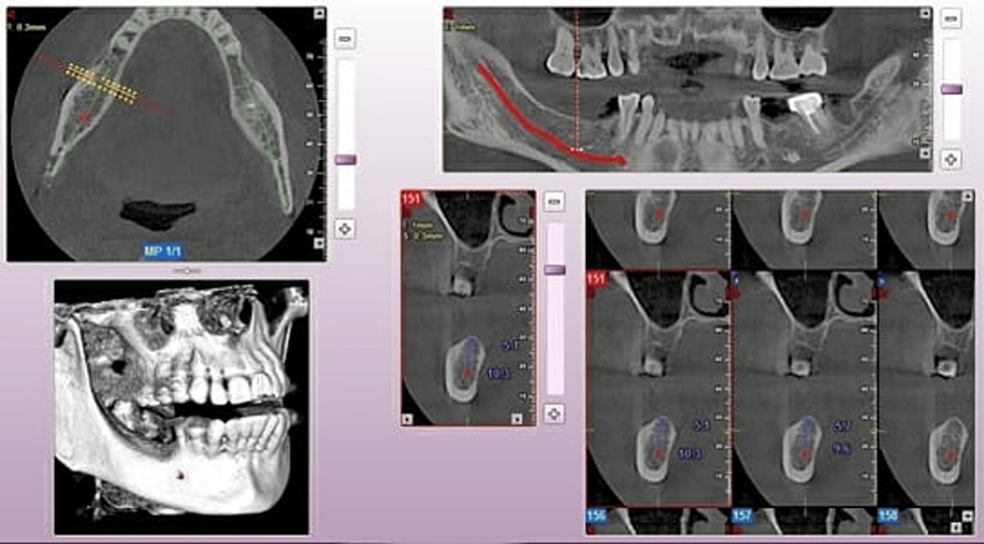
Pre-operative computer tomography (CT) image
